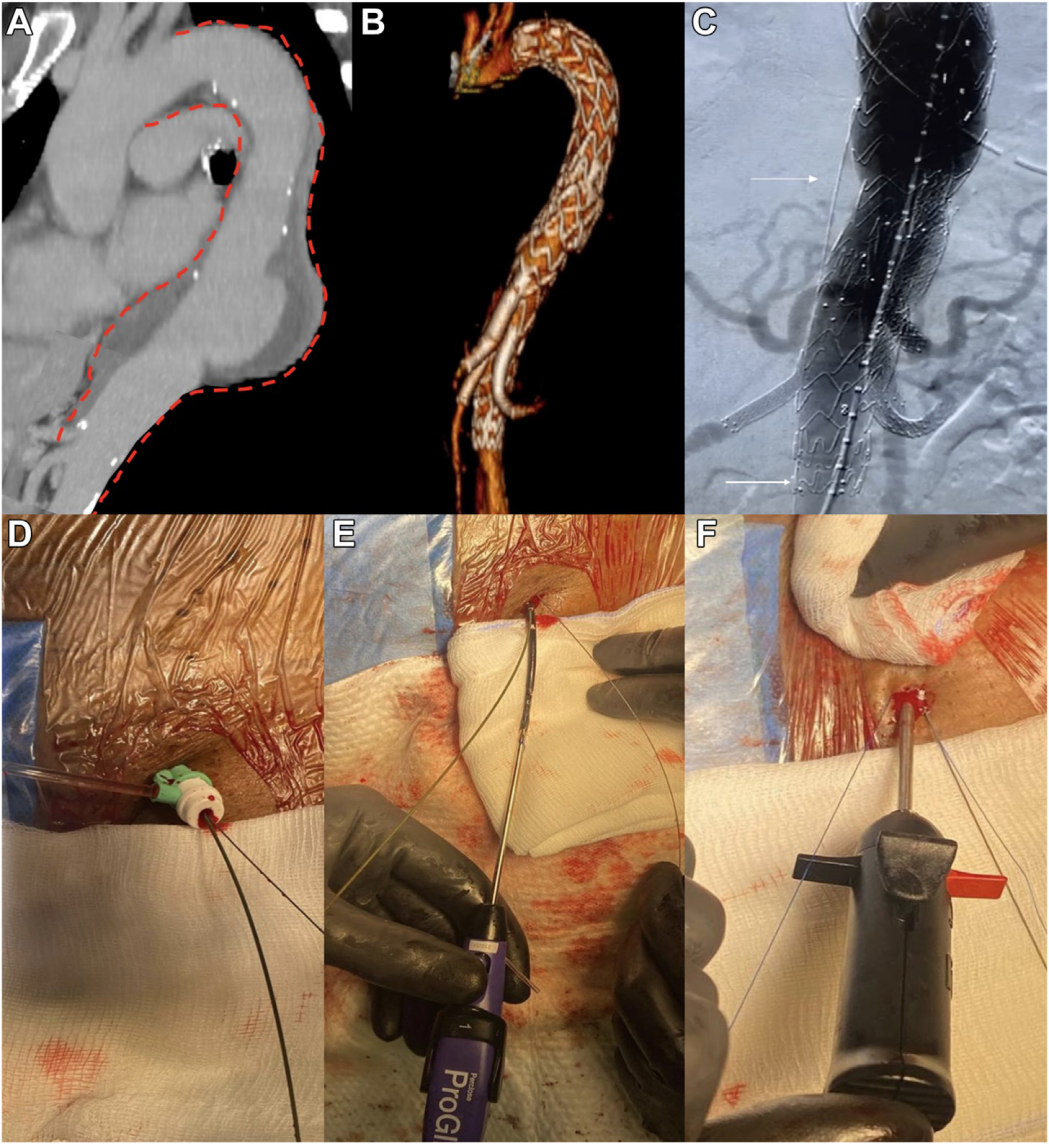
A, Computed tomography angiogram showing a thoracoabdominal aortic aneurysm (TAAA) with associated intramural hematoma. B, Postoperative computed tomography angiogram showing successful endovascular treatment with branched endovascular repair. C, Completion angiogram showing correct positioning of the endografts and bridging stents, with the V-18 guidewire (*white arrow*) left in the aneurysm sac, entering from the distal edge of the endograft. D-F, Percutaneous closure of left femoral artery access, with the V-18 guidewire left in place.
